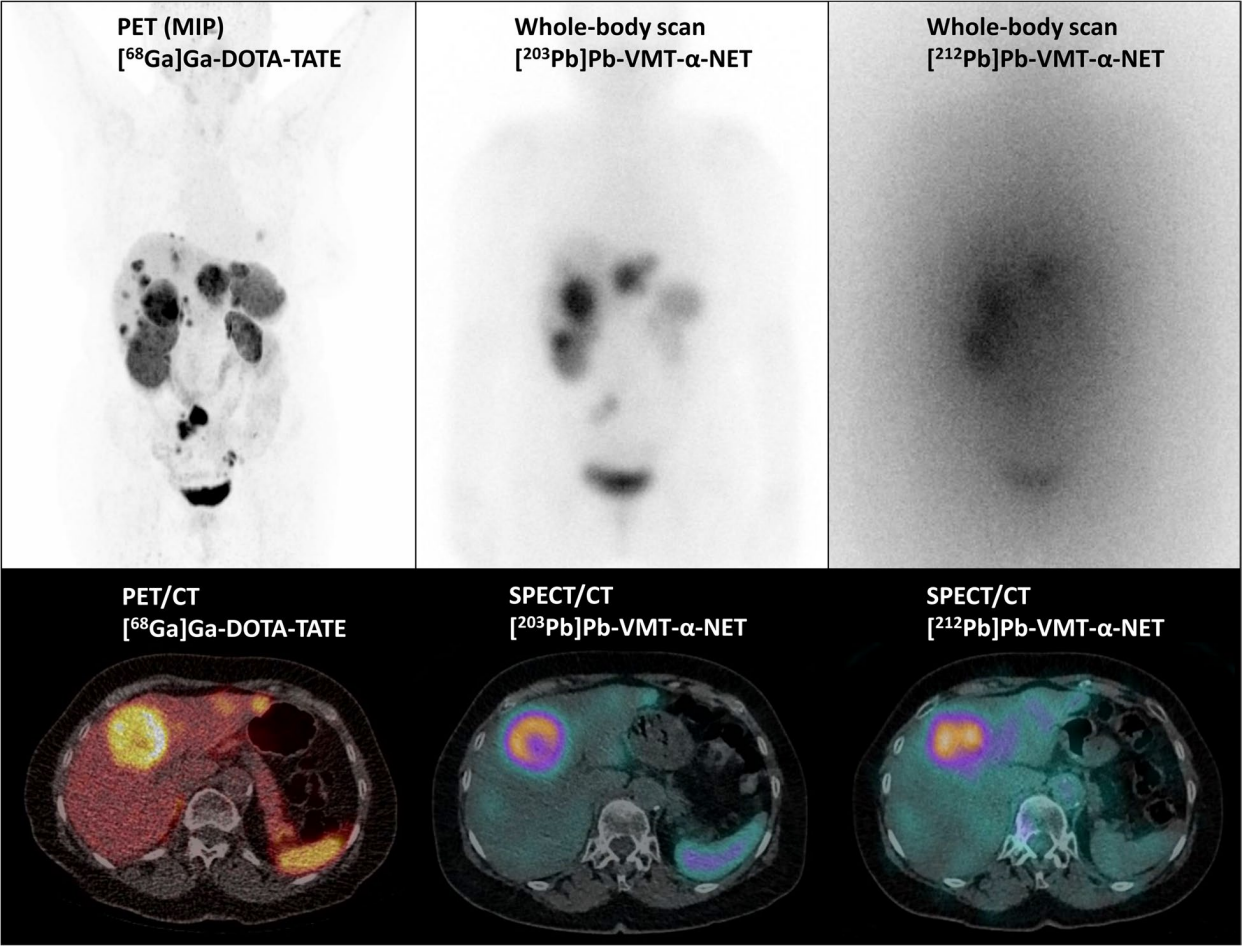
Transversal slice and maximum intensity projection (MIP) of [^68^ Ga]Ga-DOTATATE PET/CT imaging of Patient No. 9 with GEP NET G1 (left column). Whole body scans and corresponding transversal slices of pre-treatment imaging 2.5 h post-injection of 243 MBq [^203^Pb]Pb-VMT-α-NET (center column) and post-treatment imaging at 2.5 h after administration of 86 MBq [^212^Pb]Pb-VMT-α-NET (right column). The scintigraphic images showed a high accumulation of [^203/212^Pb]Pb-VMT-α-NET in liver metastases, comparable to the [^68^ Ga]Ga-DOTATATE PET/CT images
